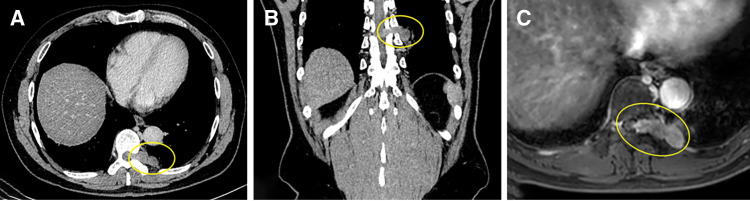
(A) Axial and (B) coronal contrast-enhanced CT identified a 20-mm dumbbell-shaped mass protruding from the left T9 intervertebral foramen into the spinal canal. (C) MRI revealed a dumbbell-shaped tumor originating from the left T9 intervertebral foramen, suggesting possible extension into the intradural space (yellow circles indicate the tumor).
